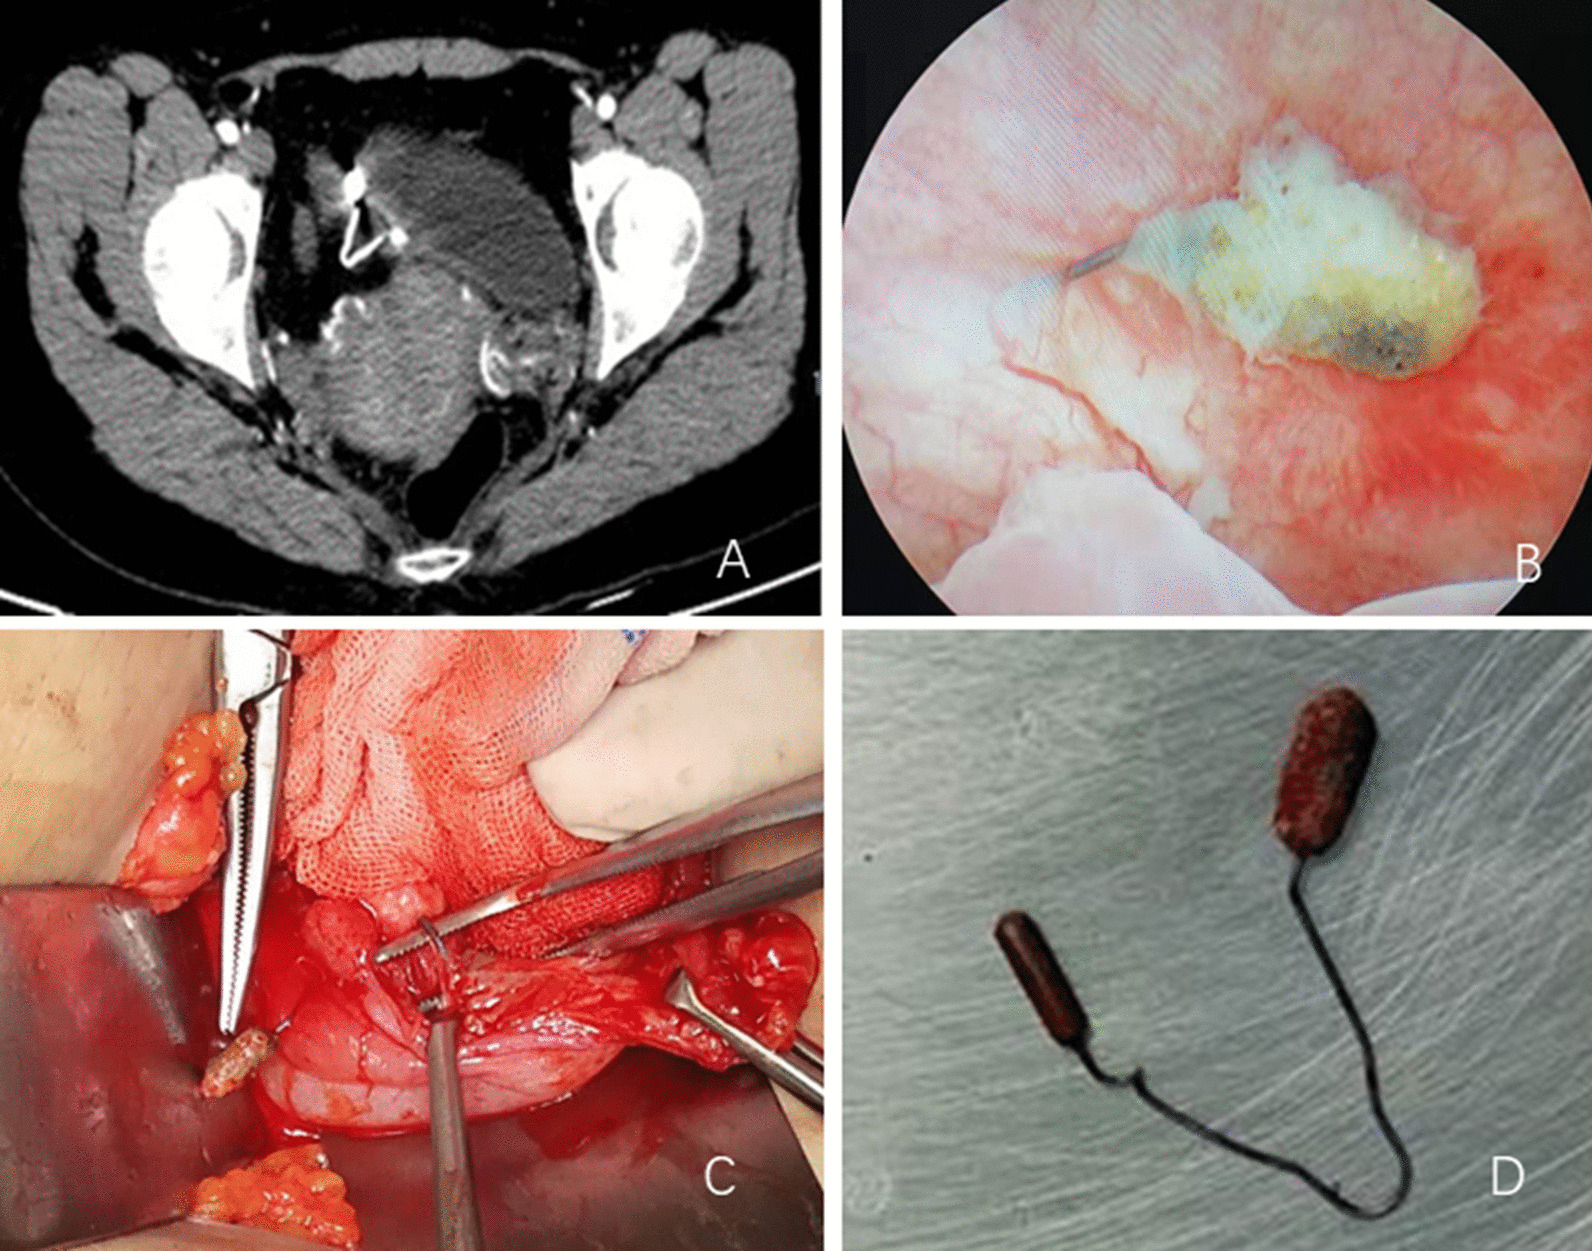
IUD of case NO.1 (a: CT scan indicating a triangular foreign body penetrated the bladder wall. b: Cystoscopy showed a strip of metal substance penetrated through the bladder wall, and the surface was covered with stones. c: Intraoperative photograph showed that a branch of the IUD passed through the bladder wall. d: The removed IUD.)
